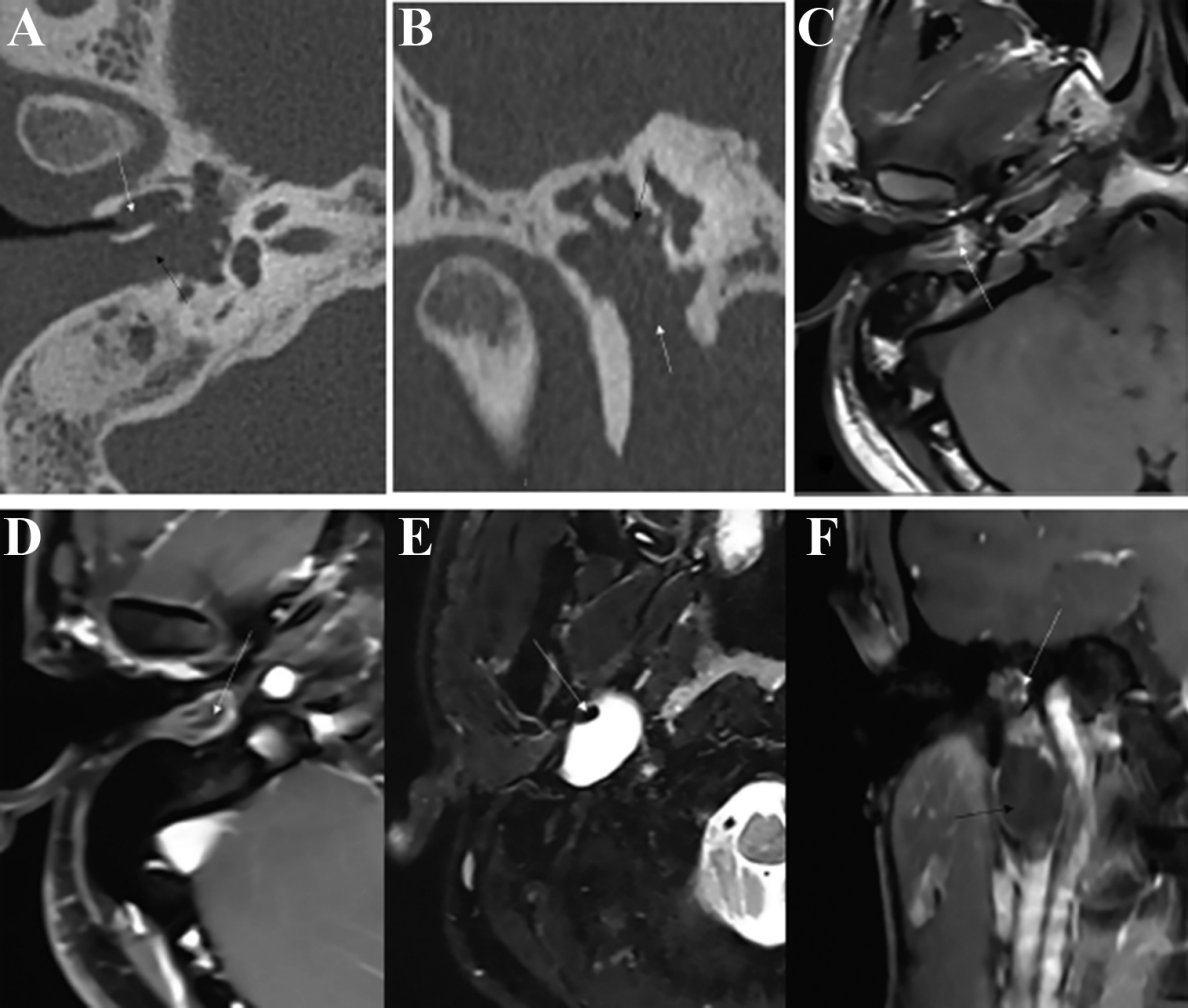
Ear malformation with MET, patient No. 8. Teratoma involved the tympanum, parapharyngeal space, and space between the posterior wall of the EAC and the mastoid, which had the appearance of two parallel bony canals. (A), axial CT imaging, stenosis of the EAC with soft tissue (white arrow), teratoma involving the space between the posterior wall of the EAC and the mastoid (black arrow). (B), Coronal MPR of the long process of the incus (black arrow); the long process of the incus was partly eroded, and the inferior wall of the tympanum (white arrow) was expanded and destroyed. (C), Axial T1WI imaging. MET demonstrated heterogeneous hyperintensity with little isointensity. (D), Axial fat-suppressed T1WI enhancement. MET showed moderate heterogeneous enhancement, and the fat tissue showed hypointensity without enhancement (white arrow) and a sandwich sign. (E), Axial fat-suppressed T2WI: Part of the MET in the parapharyngeal space was a cyst with a fat nodule (white arrow). (F), coronal fat-suppressed T1WI enhancement. Part of the MET in the tympanum showed moderate heterogeneous enhancement (white arrow), and part of the MET in the parapharyngeal space (cyst) showed no enhancement (black arrow).
